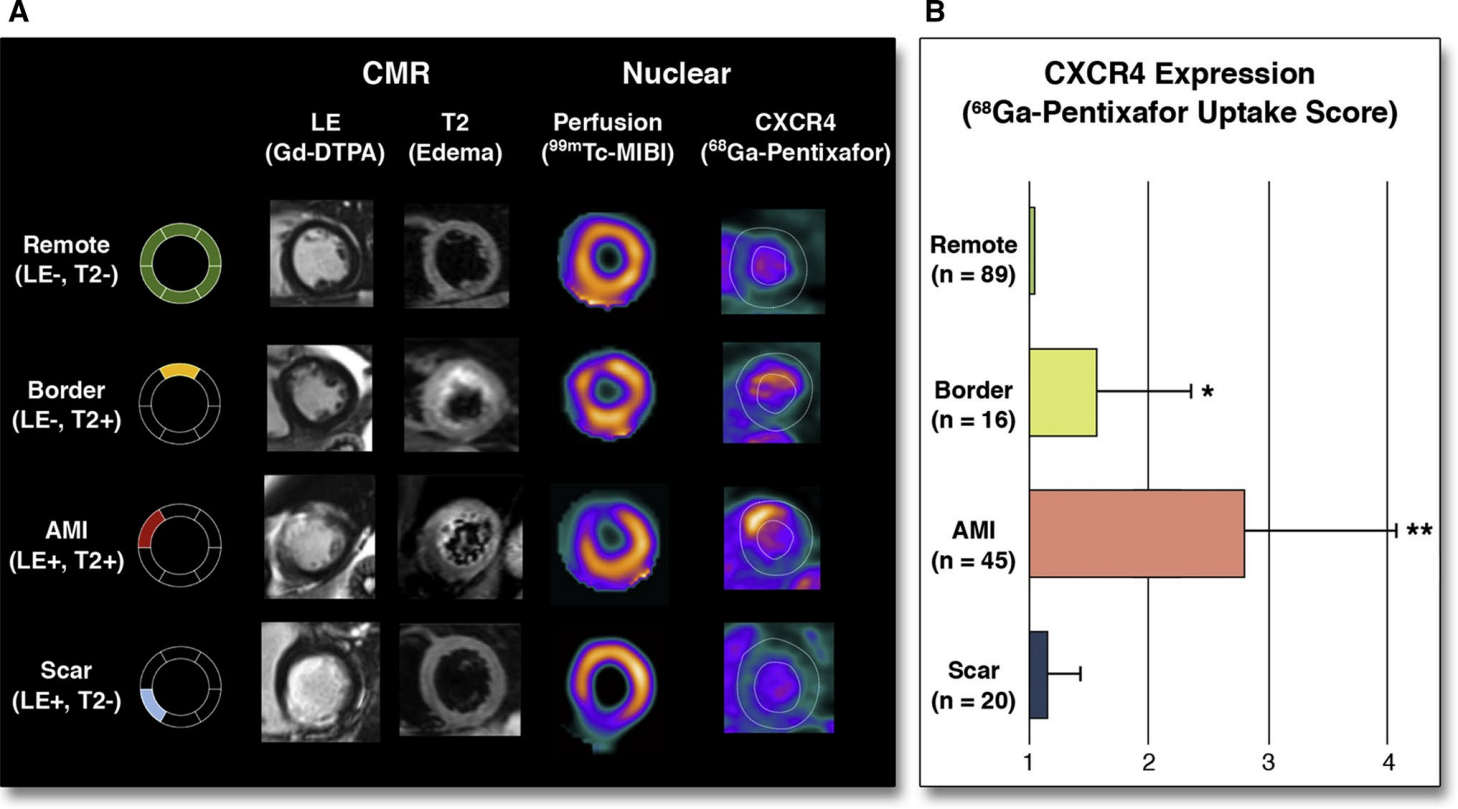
Uptake of 68 Ga pentixafor in patients after acute ST-segment elevation myocardial infarction indicating various levels of CXCR4 expression in myocardial segments with different patterns of myocardial injury as defined by the presence (+) or absence (−) of gadolinium-diethylenetriamine pentaacetic acid (DTPA) late enhancement (LE) or edema on T2-sequences (T2) at cardiac magnetic resonance imaging. a Representative short-axis slices characterizing four different types of segments. b Results of segmental pentixafor uptake score in respective segment types. p* < 0.05 versus remote; *p* < 0.05 versus all others. *HLA* horizontal long axis, *SA* short axis [139]
